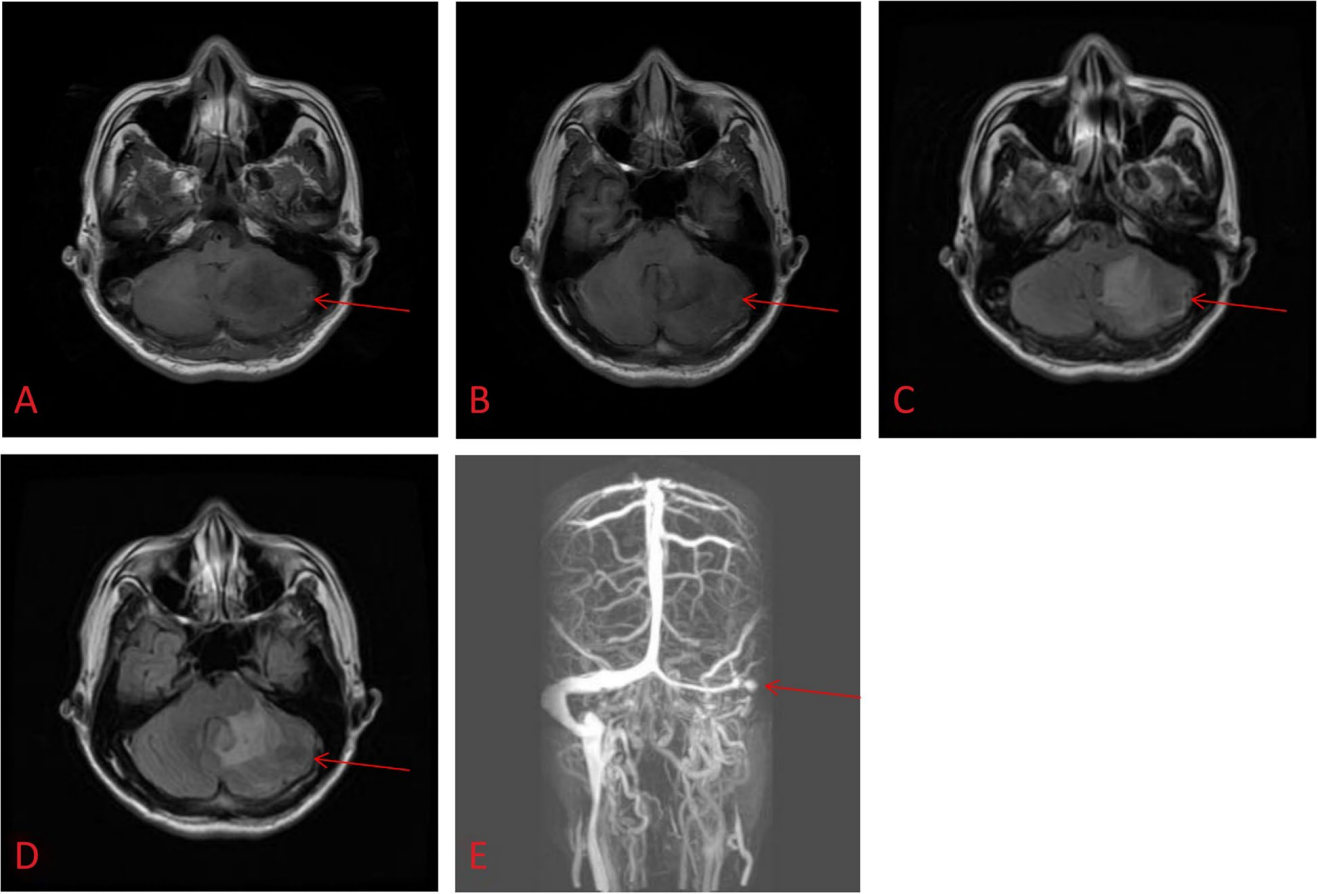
Cerebral MRI showed abnormal signal in left cerebellar hemisphere, vermis cerebelli, pontibrachium(A, B: T1W1 showed hypointensity, C: T2WI showed hyperintensity, D: Flair showed hyperintensity); MRV showed left sigmoid sinus was indistinct(E)
